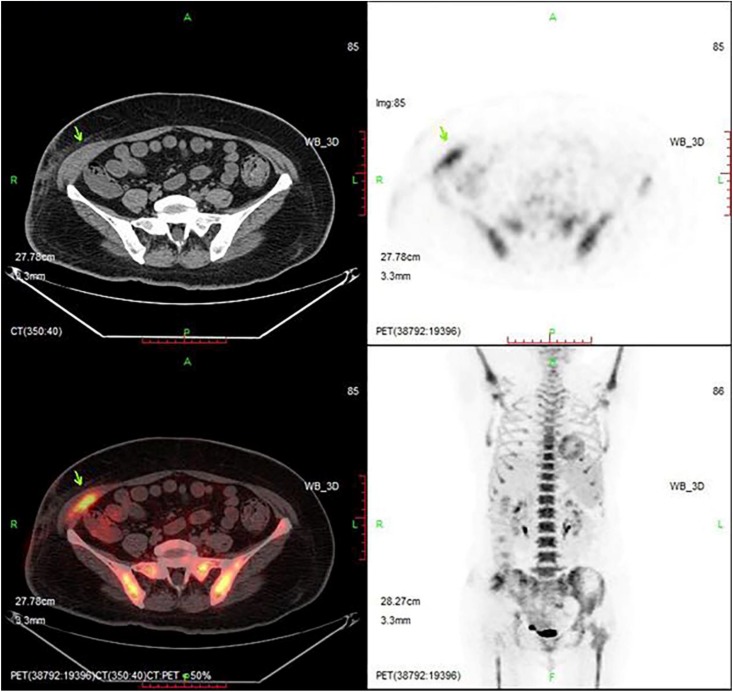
PET/CT imaging after CAR-T cell infusion. The lesion is significantly smaller than before. The position indicated by the arrow is the location of the lesion.
